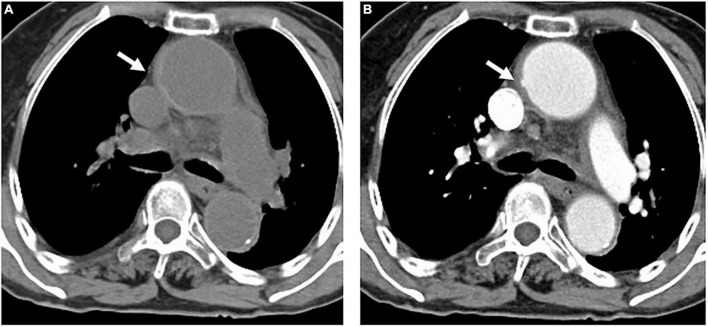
An 84-year-old woman with TAIMH and tiny ULP. A precontrast CT scan showed circular high attenuation aortic wall thickening (5 mm) (arrow) involving the ascending aorta (49 mm) (A). A tiny ULP (arrow) was observed in the greater curvature of the ascending aorta (B). Follow-up CT was not performed and the patient suffered from sudden death on the fourth day of admission. CT, computerized tomography; TAIMH, type A aortic intramural hematoma; ULP, ulcer-like projection.
